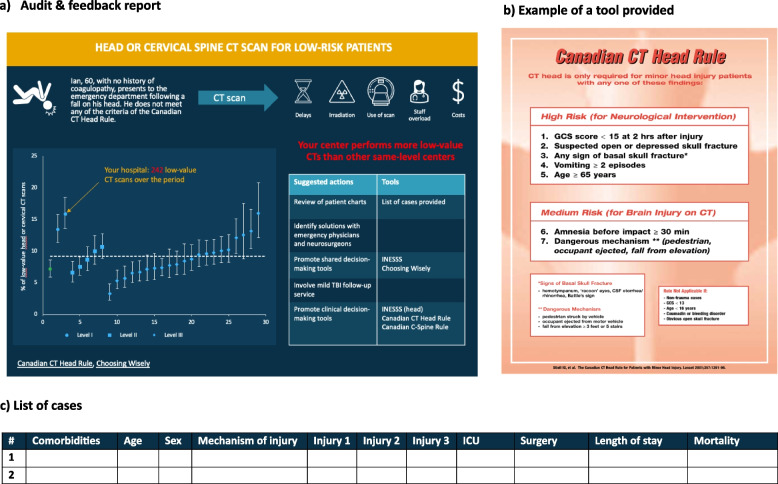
Example of initial intervention prototype for head or cervical spine CT in low-risk patients
